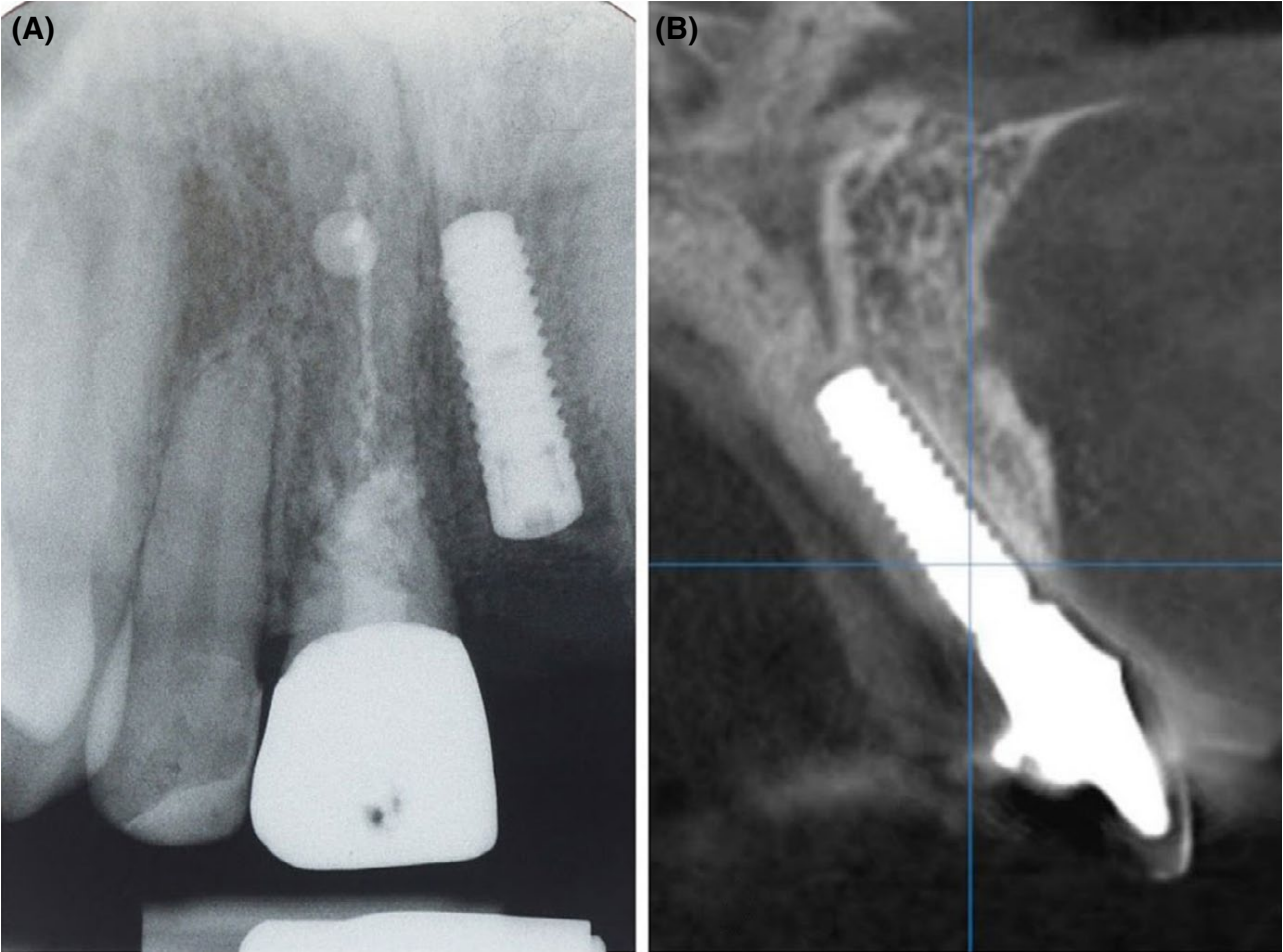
X‐ray image immediately after #21 implant placement (A) and CBCT scan in the region of #21 1 year postoperatively (implant #21 with provisional crown) (B)
